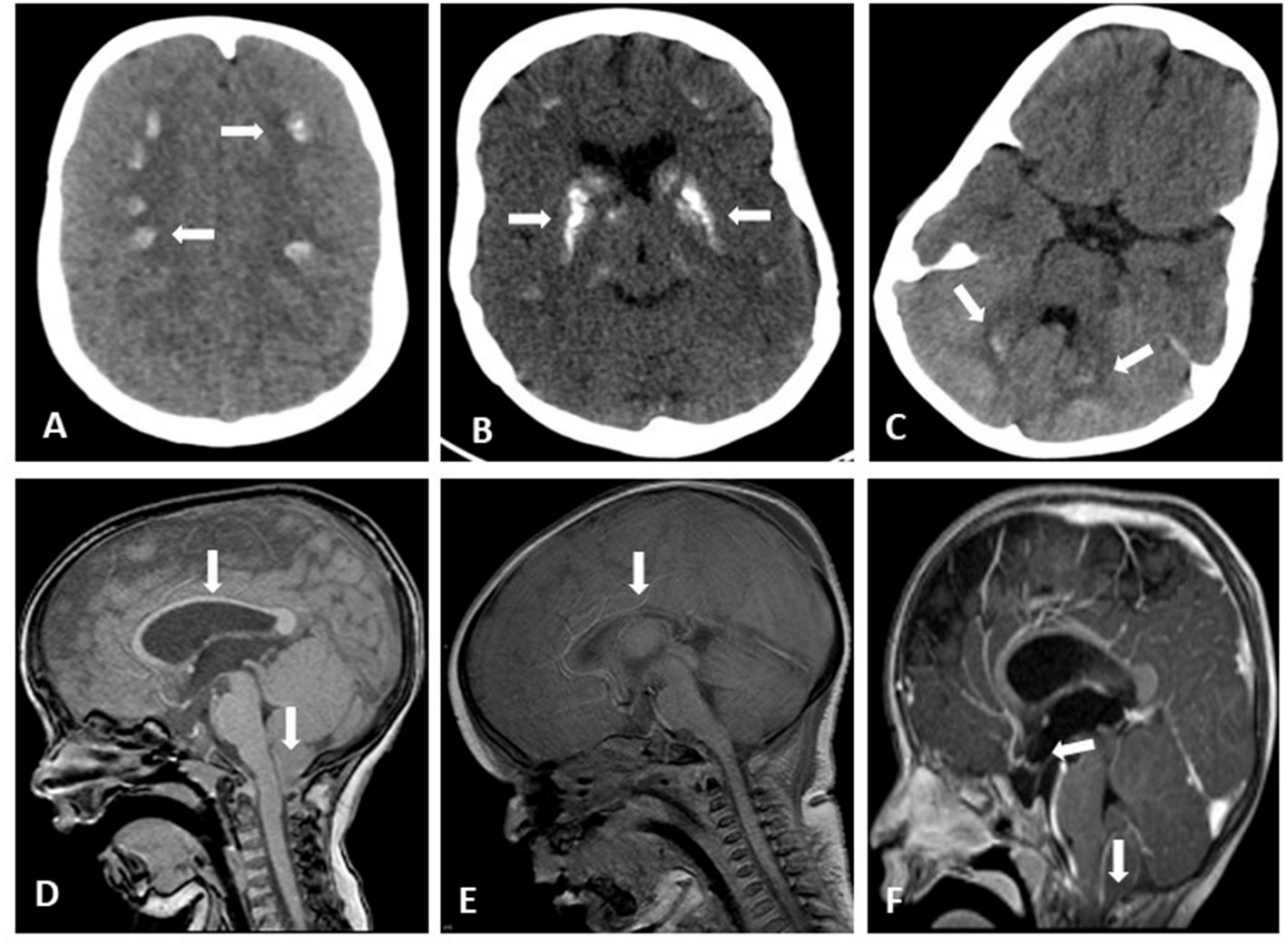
Brain imaging of HRD patients. Axial brain CT without contrast showing bilateral frontoparietal calcifications (A), bilateral basal ganglia coarse calcifications (B) small bilateral Dentate Nucleus calcifications (C); Sagittal brain MRI T1 without contrast showing Chiari Malformation and thin Corpus Callosum (D) considerable tightening of Corpus Callosum (E); Sagittal brain MRI T1 with gadolinium showing drooping of cervical tonsils trough the foramen magnum and pituitary gland flattening (F).
